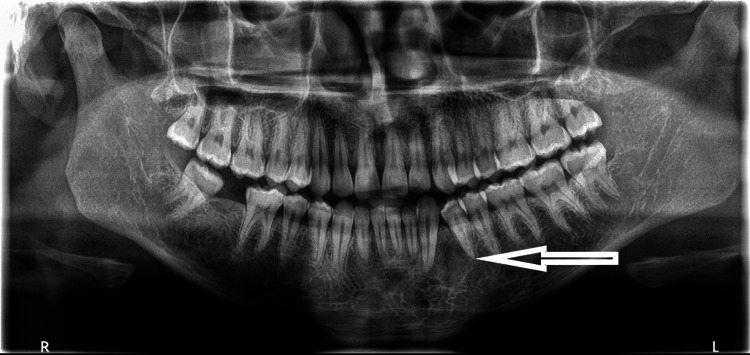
Pre-operative OPG, ill-defined multi-locular radiolucency between tooth #33 and #34, pushing the roots medially and distally, respectively, without causing root resorption. The white arrow is pointing towards the lesion. OPG: orthopantomogram
